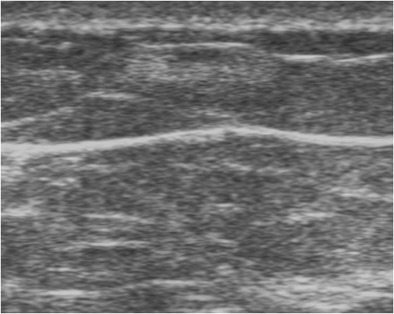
Haemangioma in a 72-year-old woman, presenting with a slowly growing, superficial breast opacity. Ultrasound: oval-shaped, hyperechoic mass of the subcutaneous fat, parallel to the skin. Surgical resection in order to eliminate angiosarcoma: confirmation of the benign nature of the tumour
